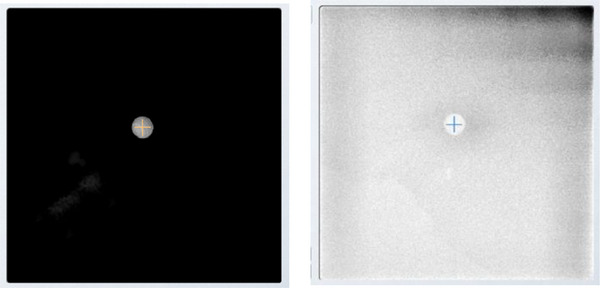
(a) DRR of the TRUFIX steel ball captured with CT before the scan; (b) TRUFIX steel ball imaged with ExacTrac.
